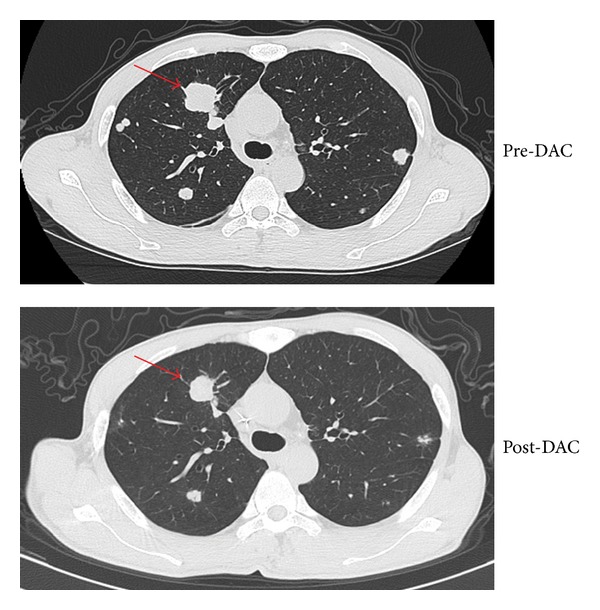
CT image for UPN 20 exhibited a partial response. A CT image showing the specific characteristics of the response of lung lesion following four cycles of decitabine treatment, assessing by the Response Evaluation Criteria in Solid Tumors (RECIST). The red arrows indicate the areas of measurable disease.
